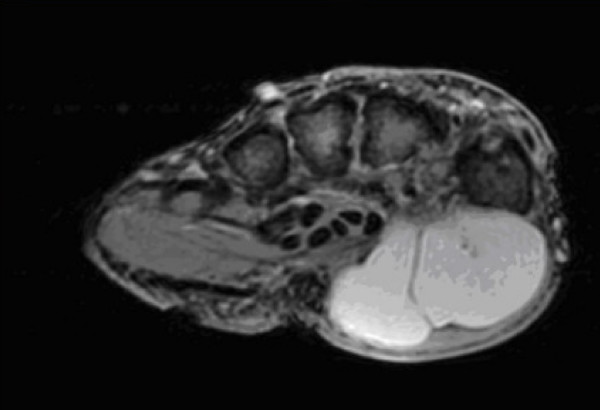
MRI appearance of thenar eminence ganglion.
